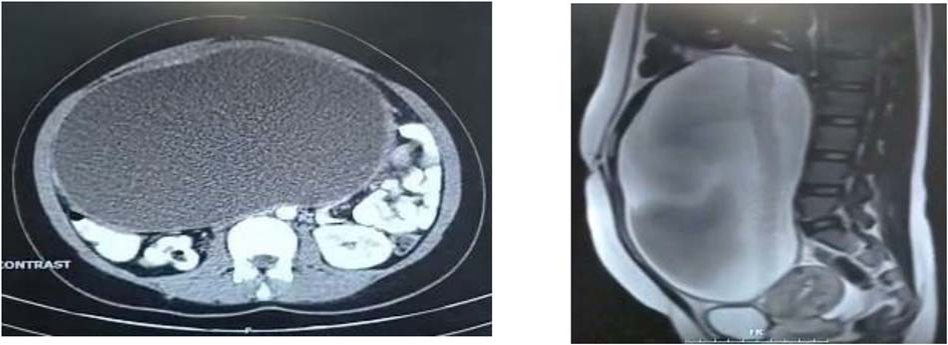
CT scan of the abdomen represents an extension of the mass in two specific views. (a) From the front of the lumbar vertebra to the abdominal wall in horizontal view. (b) Below the peritoneal cavity to the above the bladder in sagittal view.
